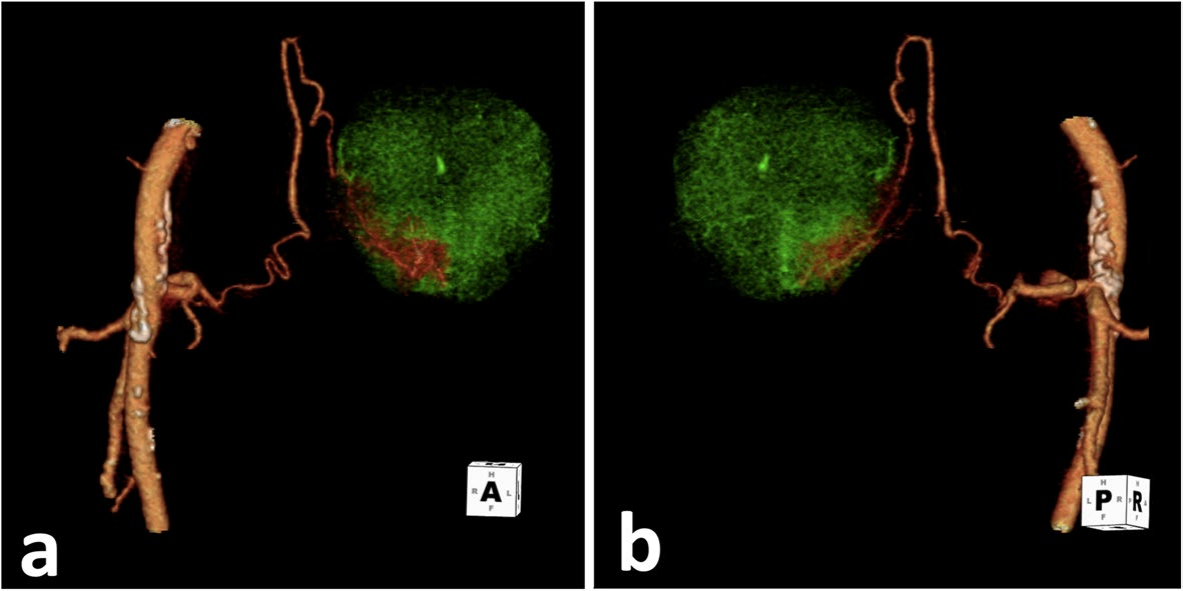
3-D volume-rendered technique (VRT) reconstruction from the pre-procedure CTA of the prostatic artery origin (a) Anterior view of the aberrant prostatic artery. b Posterior view of the trifurcation giving rise to the prostatic artery
